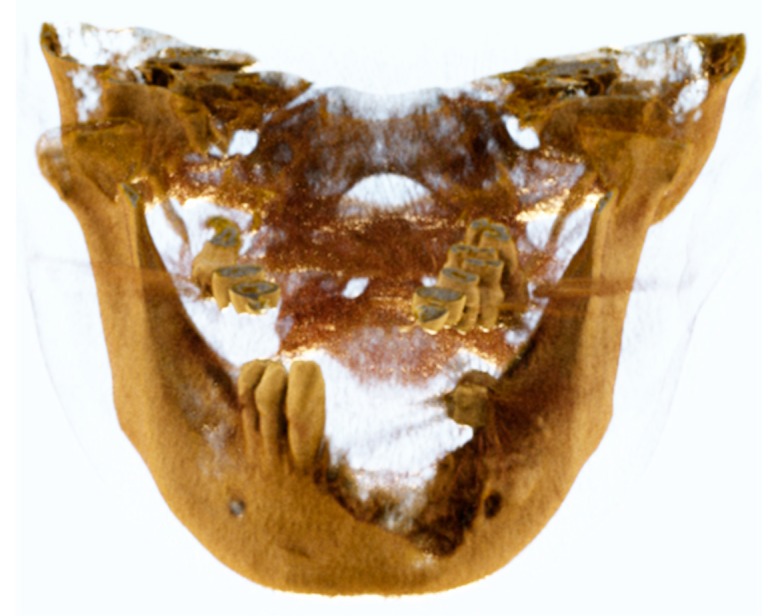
Computerized tomography (CT) scan that showed the presence of a tumor mass in the anterior region of the mandible.
